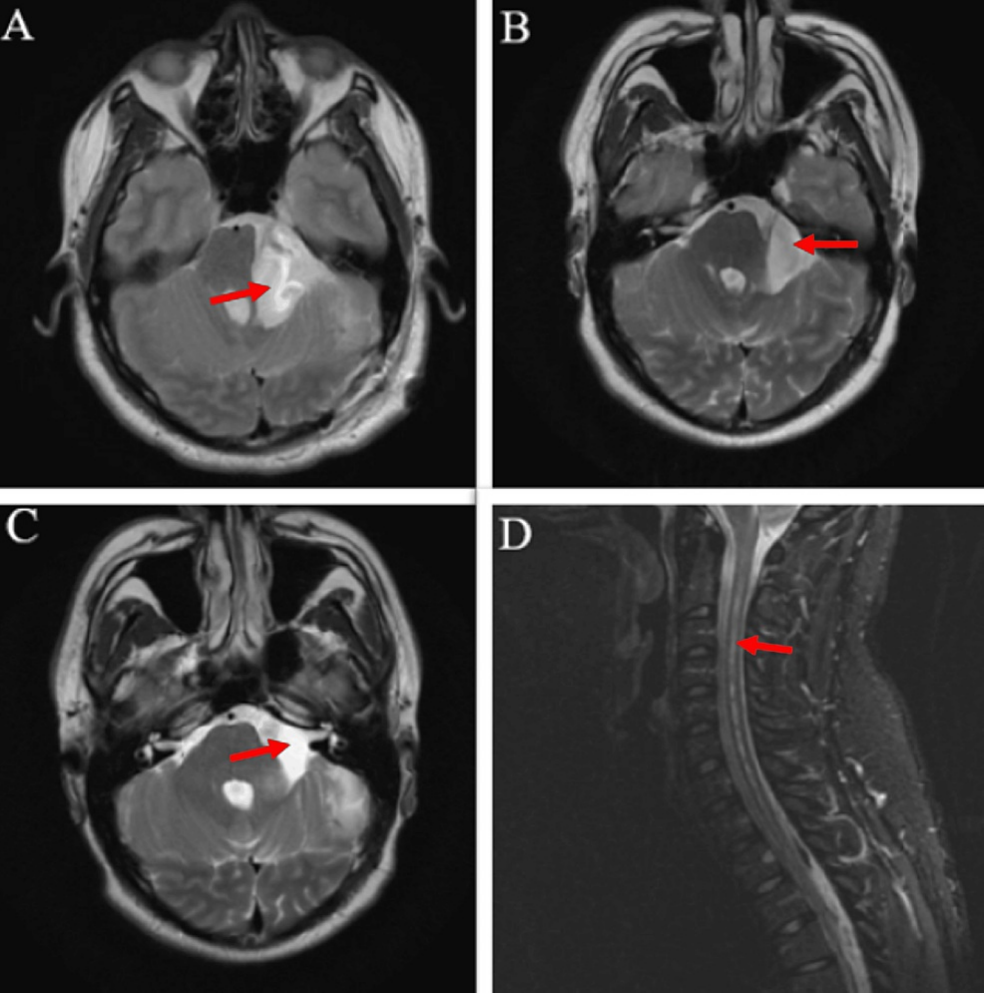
Postoperative MRI with arrows demonstrating improved PFAC, axial T2 MRIs (a) postoperative day 1; (b) six months postoperative; (c) 12 months postoperative; (d) sagittal T2 cervical MRI at 12 months postoperative, with arrow demonstrating the near-complete resolution of the syringomyelia
